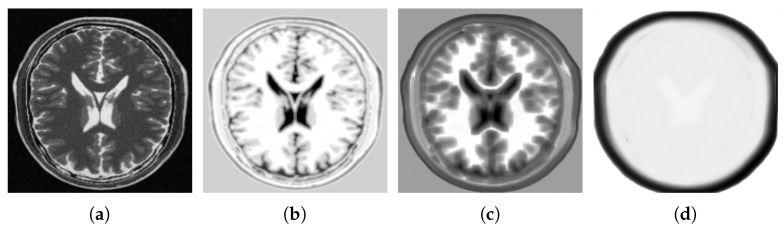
(a) A slice of T2-MRI image and it’s image representation of the embedded manifold with: (b) D=9; (c) D=49; and (d) D=225. The higher the *D*, the more blurred/general structural representation.
